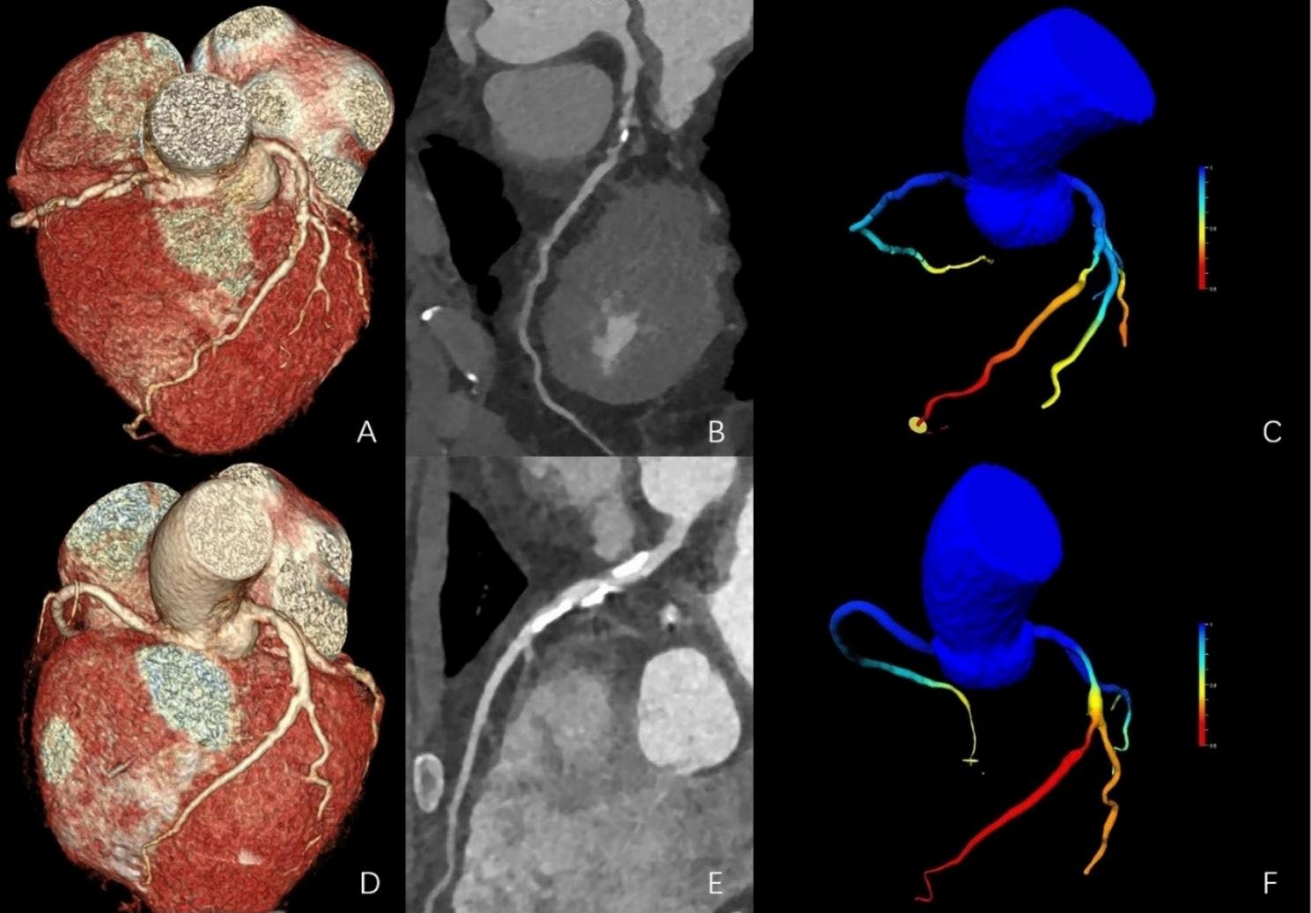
Representative case from the present study. CCTA showed severe (> 75%) stenosis in the proximal LAD with a mixture plaque of the non-calcified and calcified component and FFR_CT_ value of 0.75 in a 58-years old male with atypical chest pain (A-C). CCTA showed severe (> 75%) stenosis in proximal and middle LAD with a calcified plaque and FFR_CT_ value of 0.60 in a 55-years old female with atypical chest pain and chest tightness (D-F). Volume rending (A, D), LAD curve planner reformation (B, E), and FFR_CT_ analyses (C, F). CCTA = coronary computed tomography angiography, LAD = left anterior descending artery, FFR_CT_ = computed tomography–derived fractional flow reserve.
